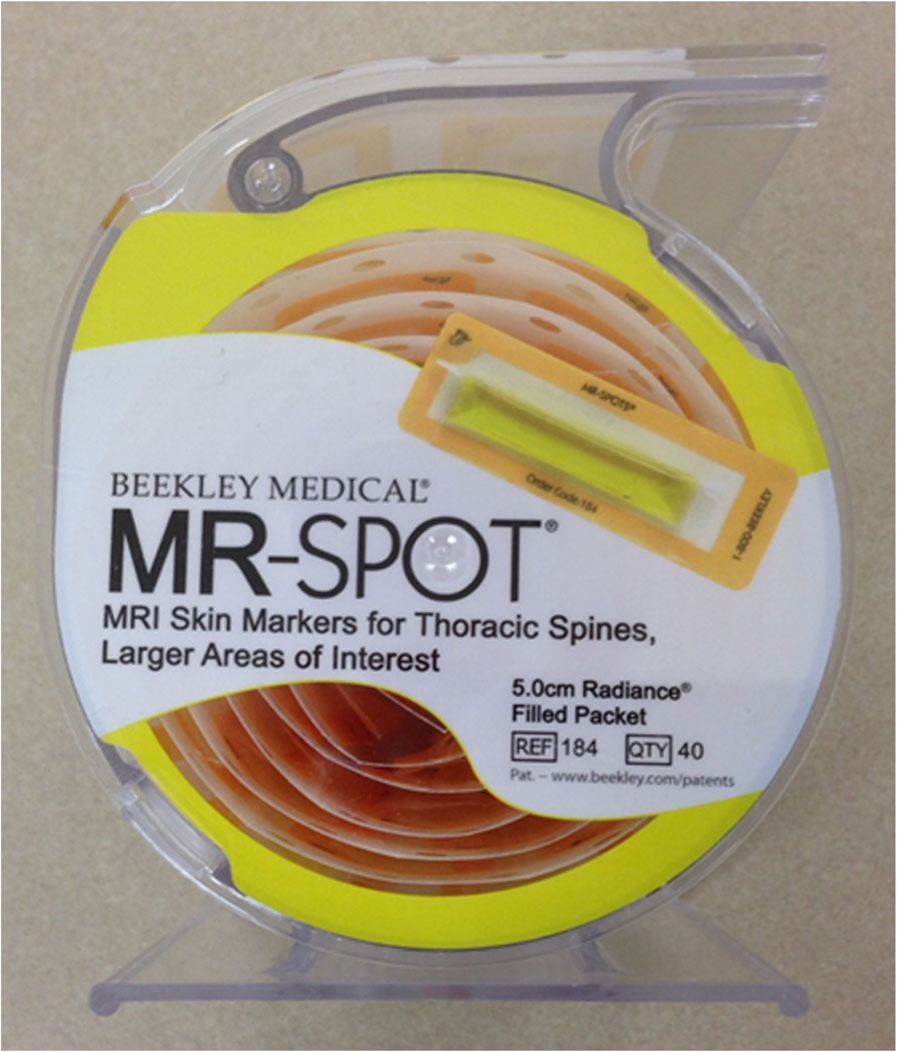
MR-SPOT MRI skin marker (Beekley Medical Corporation)
